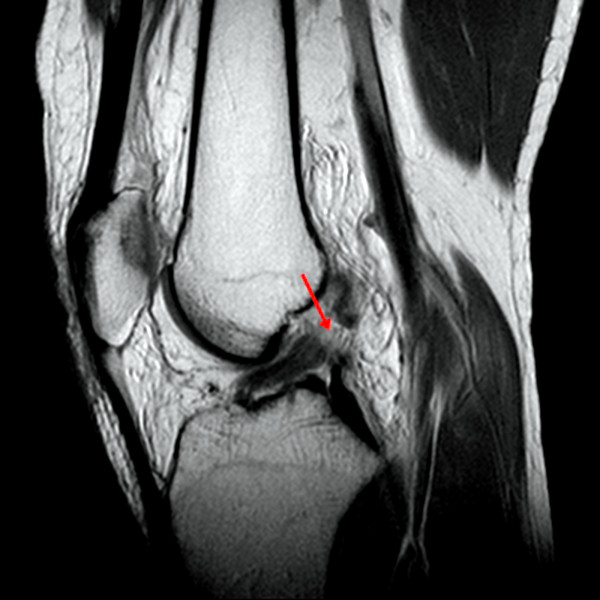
In this T2-weighted fat-suppressed sagittal image, discontinuity of anterior cruciate ligament (ALC) fibers is shown (arrow). Discontinuity is defined as a focal gap or interruption of the ACL fibers.
